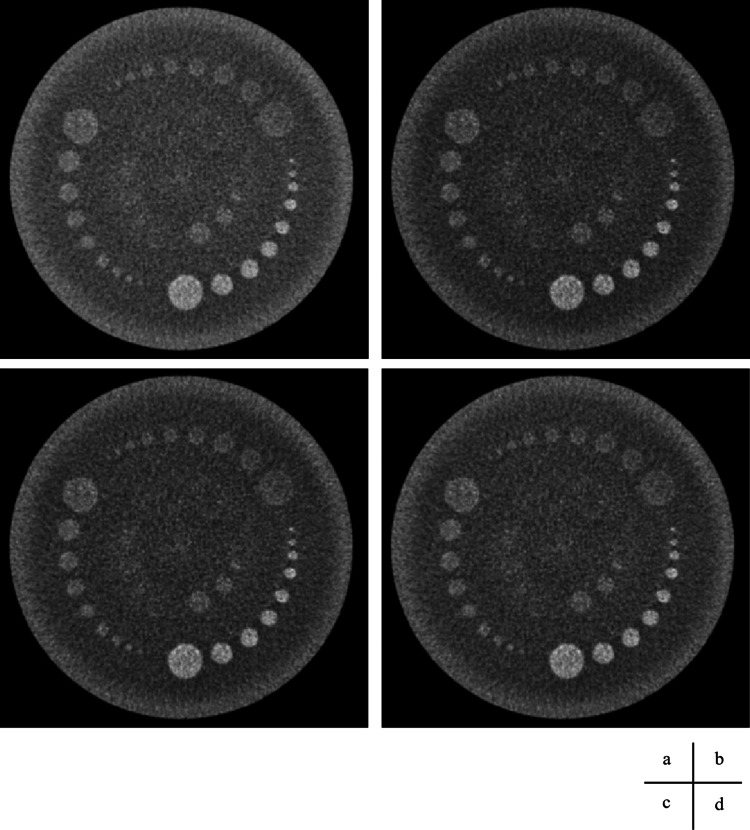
Helical scan and processed images using square transformations, displayed with a wide window. (a) Original CT image: window level (WL) = 50 Hounsfield units (HU); window width (WW) = 40 HU. (b) Square−5 image: WL = 2425 HU; WW = 3600 HU. (c) Square image: WL = 2900 HU; WW = 4000 HU. (d) Square+5 image: WL = 3425 HU; WW = 4400 HU.
